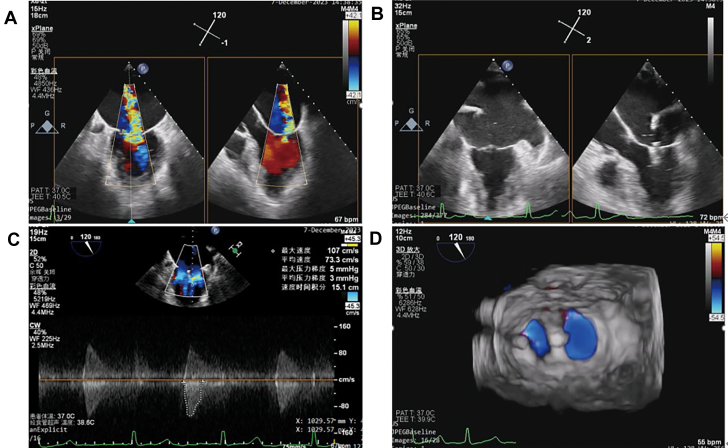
Transesophageal Echocardiography Images Obtained During the Transcatheter Mitral Valve Edge-to-Edge Repair Procedure (A) Evaluation of severe mitral regurgitation intraoperatively. (B) Successful grasping of the anterior and posterior mitral valve leaflets. (C) Measurement of mitral valve flow velocity and mean pressure gradient. (D) Visualization of the tissue bridge structure and residual regurgitation.
